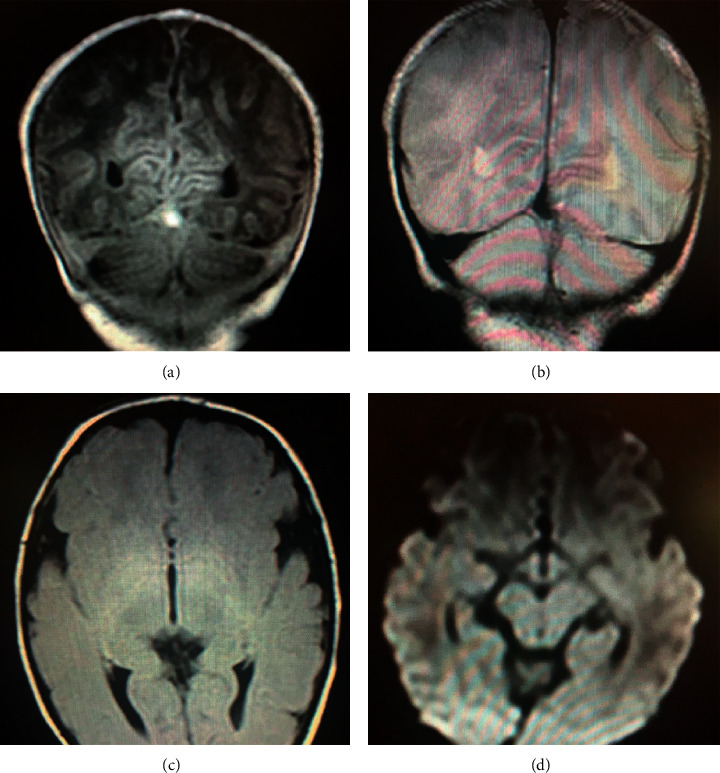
Coronal view of the brain. (a) T1-weighted, (b) T2-weighted (c), flair, and (d) diffusion-weighted. No abnormalities were detected.
